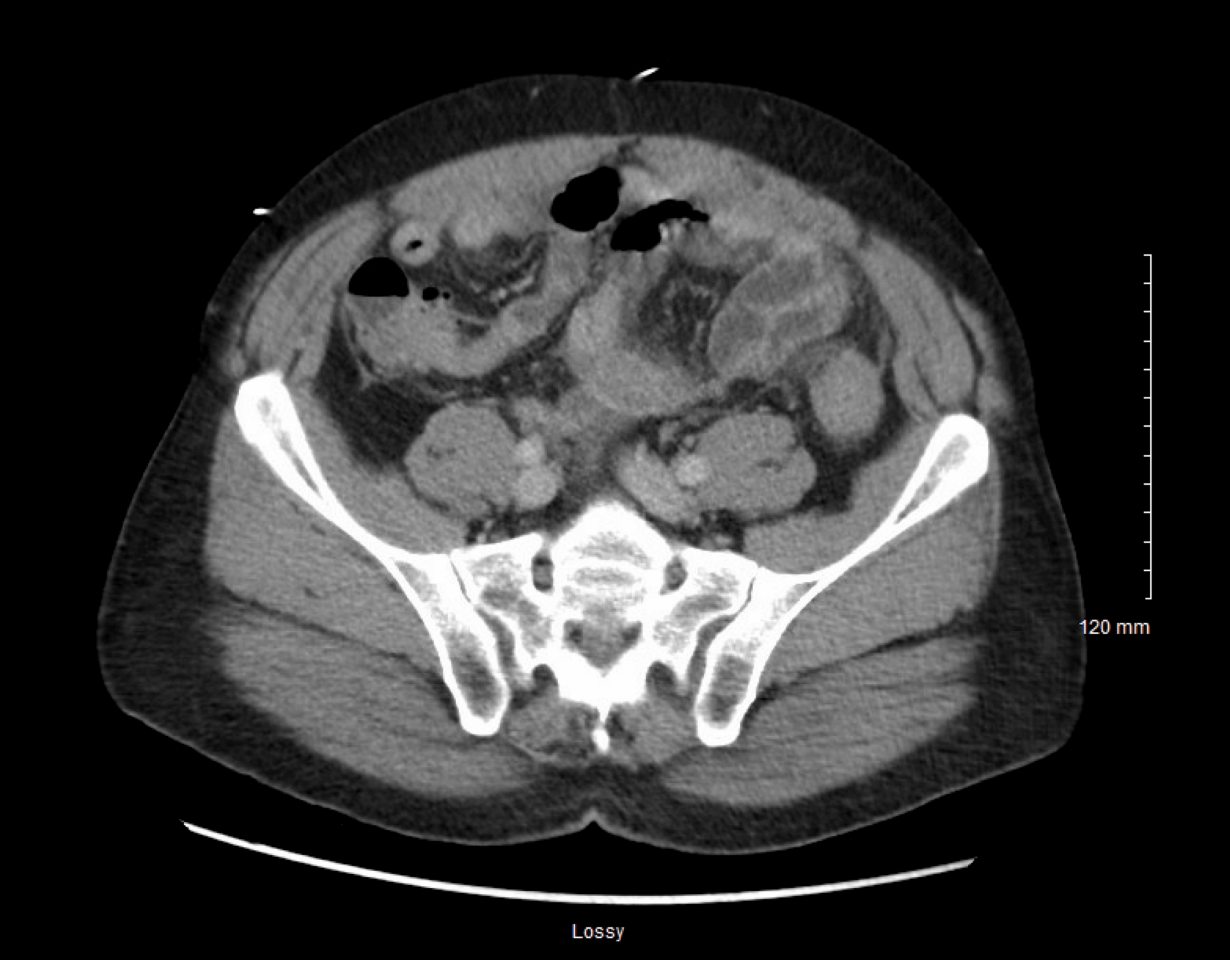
CT abdomen axial view This figure demonstrates moderate free intraperitoneal fluid within the pelvis and left paracolic gutter, without evidence of free air. The presence of free fluid in the absence of perforation supports the diagnosis of intestinal AE and helps distinguish it from hollow viscus perforation or inflammatory intra-abdominal processes.
